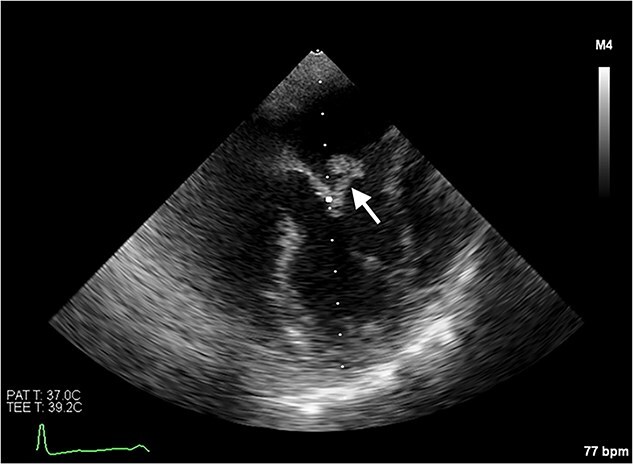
Transoesophageal echocardiogram showing the pedunculated mass on the anterior mitral valve leaflet.
